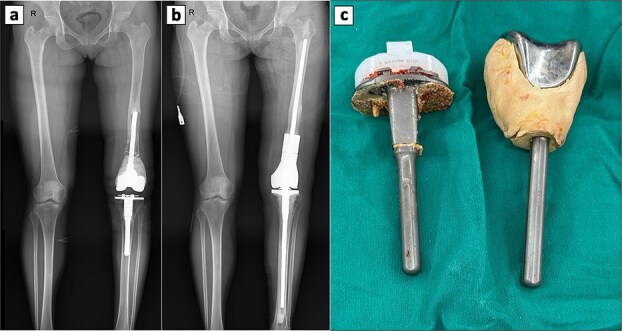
Perioperative radiographic imaging and retrieved prosthetic components following revision total knee arthroplasty. (a) Anteroposterior radiograph after primary total knee replacement, showing the femoral prosthesis loosening. (b) Anteroposterior radiograph after revision total knee replacement. (c) Retrieved prosthesis from the primary total knee arthroplasty, including femoral and tibial components.
